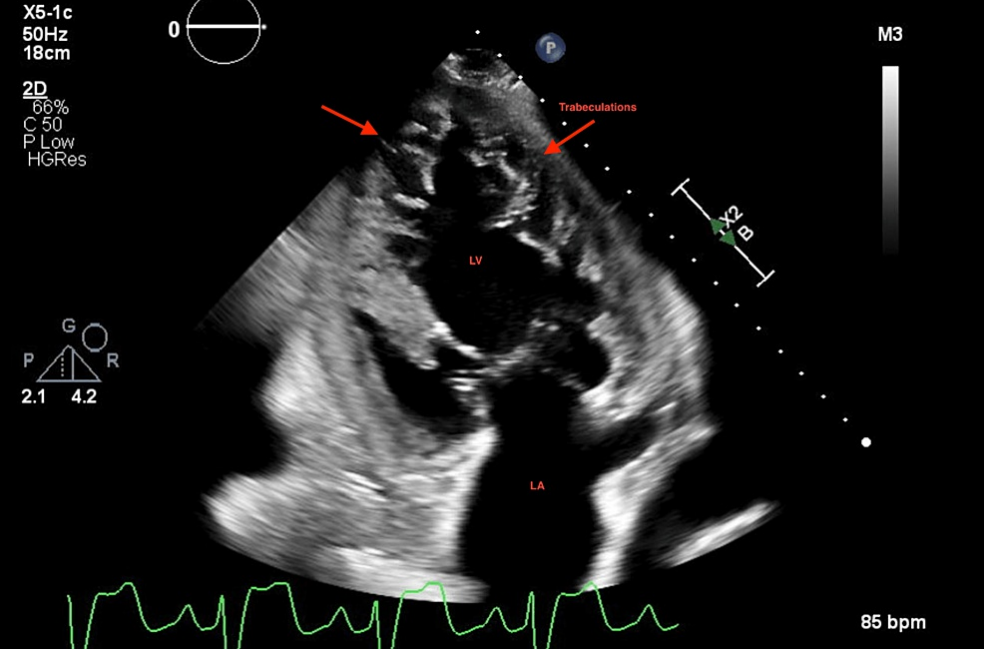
2D transthoracic echocardiogram (two-chamber view) of the patient with pronounced trabeculations of the left ventricle (red arrows)
